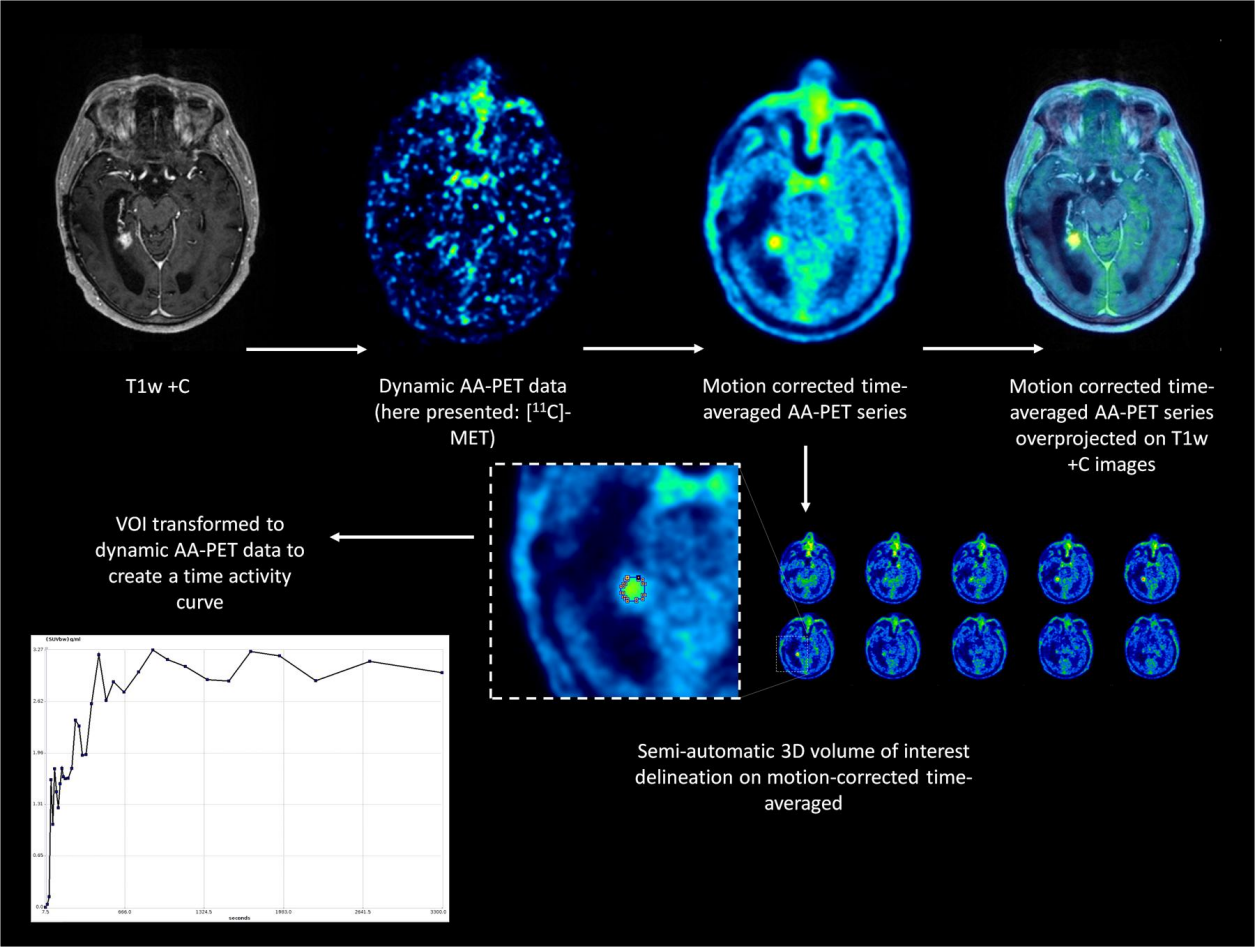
Dynamic AA-PET analysis methodology. Following the intravenous injection of either [^11^C]MET or [^1^⁸F]FET, dynamic amino-acid PET data were acquired continuously for 60 min. Motion correction and co-registration of PET with anatomical T1-weighted MRI were performed using PMOD software. Semi-automatic 3D VOI delineation (hot 3D method) was used to segment the new contrast-enhancing lesion, guided by the fused PET-MRI dataset. A reference VOI was positioned over contralateral normal-appearing brain tissue to calculate the tumor-to-brain ratio (TBR). From the dynamic data, both static (TBR_mean_ and TBR_max_) and dynamic (time to peak, TTP) PET parameters were extracted for subsequent statistical analyses.
